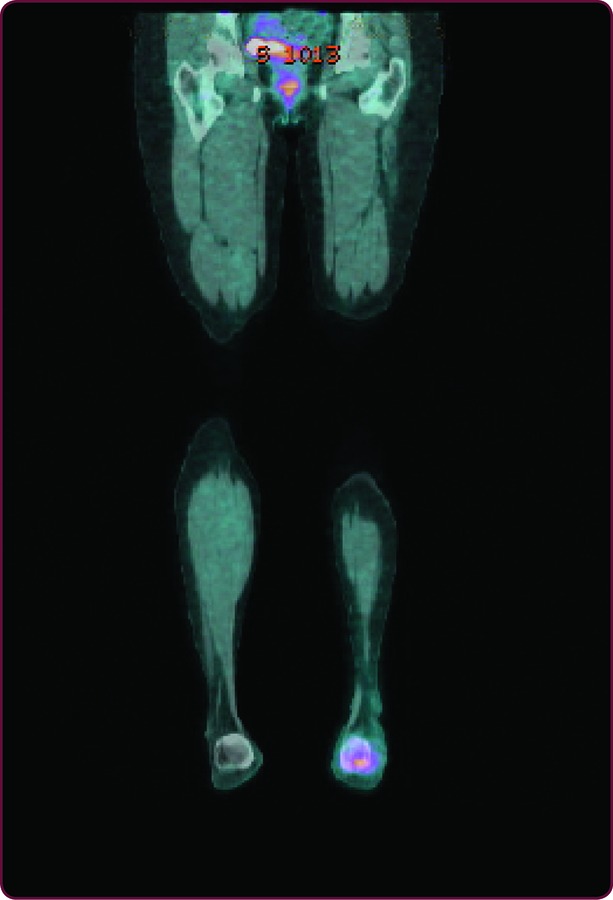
This staging PET-CT scan shows a moderate hypermetabolic primary malignant lesion in the left calcaneus.
